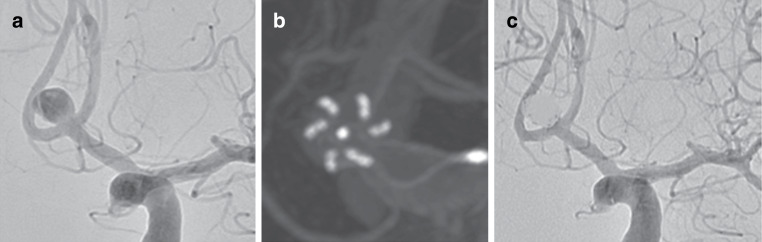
A patient with an unruptured aneurysm of the AcomA artery (a). A vaso CT reconstruction performed intraoperatively demonstrates the position of the pCONUS2 (4 × 15 × 6 mm) providing complete coverage at the neck of the aneurysm (b). Complete occlusion (mRRC 1) seen at the end of the procedure (c)
